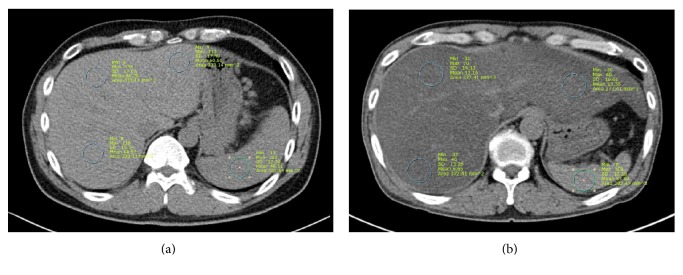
Unenhanced CT images from two patients with acute pancreatitis. (a) 54-year-old man with normal liver attenuation (60 HU) compared with spleen (46 HU). (b) 46-year-old man with severe fatty liver. Mean liver attenuation (13 HU) was significantly lower than spleen attenuation (54 HU).
